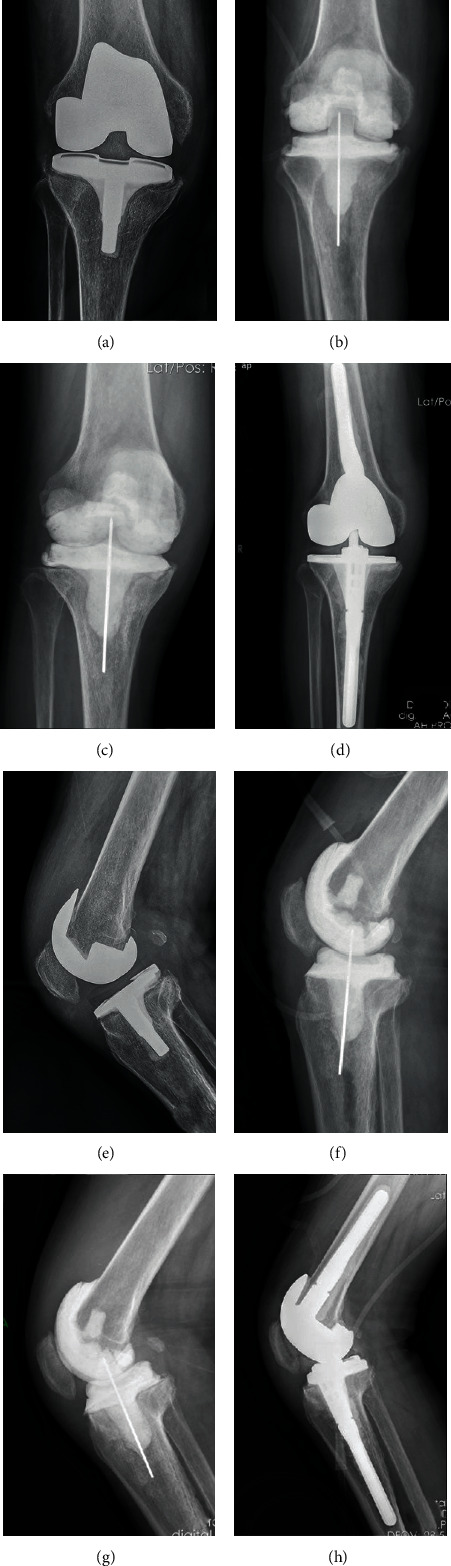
(a, e) X-ray films of periprosthetic joint infections. (b, f) X-ray films immediately after insertion of 3D printing-assisted articulating spacer. (c, g) Eight weeks later, X-ray films showed no obvious bone loss. (d, h) X-ray films immediately after the second-stage revision.
